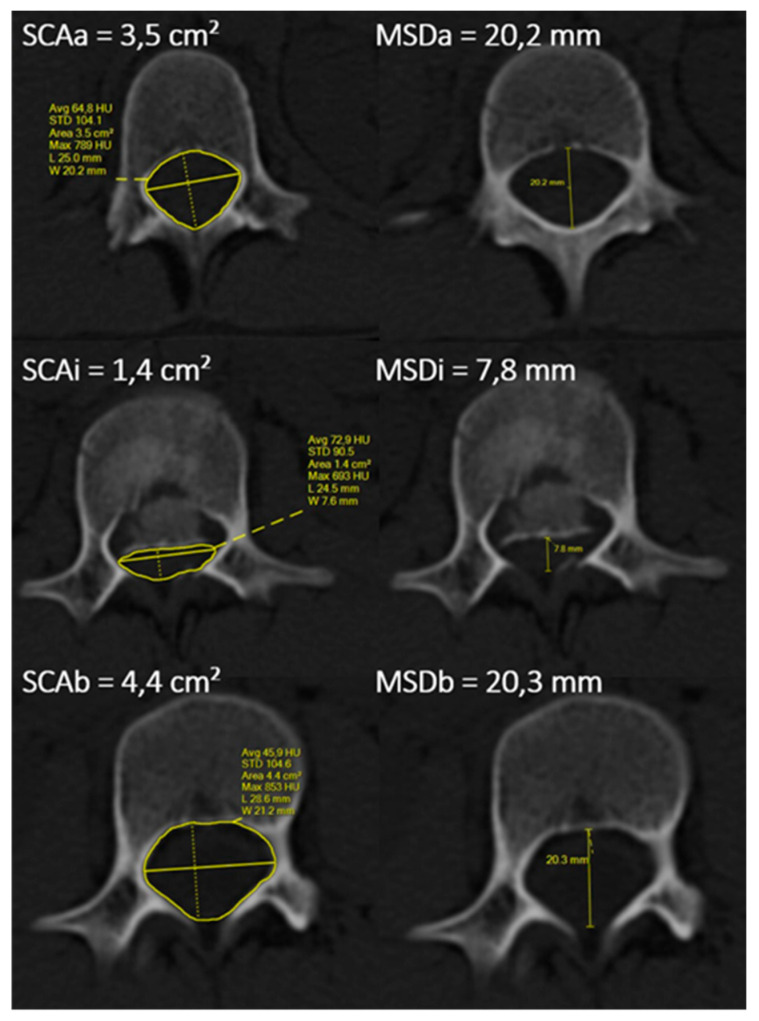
The analysis of SCA (the yellow lines encircling the inner boundaries of the spinal canal) and MSD (the yellow median plane lines) parameters is presented. Standard CT transverse plane projections (bone window) were used. SCAa/MSDa indicates the level (vertebra) above the fracture, SCAi/MSDi describes the fractured vertebra, and SCAb/MSDb designates the level below the fracture.
